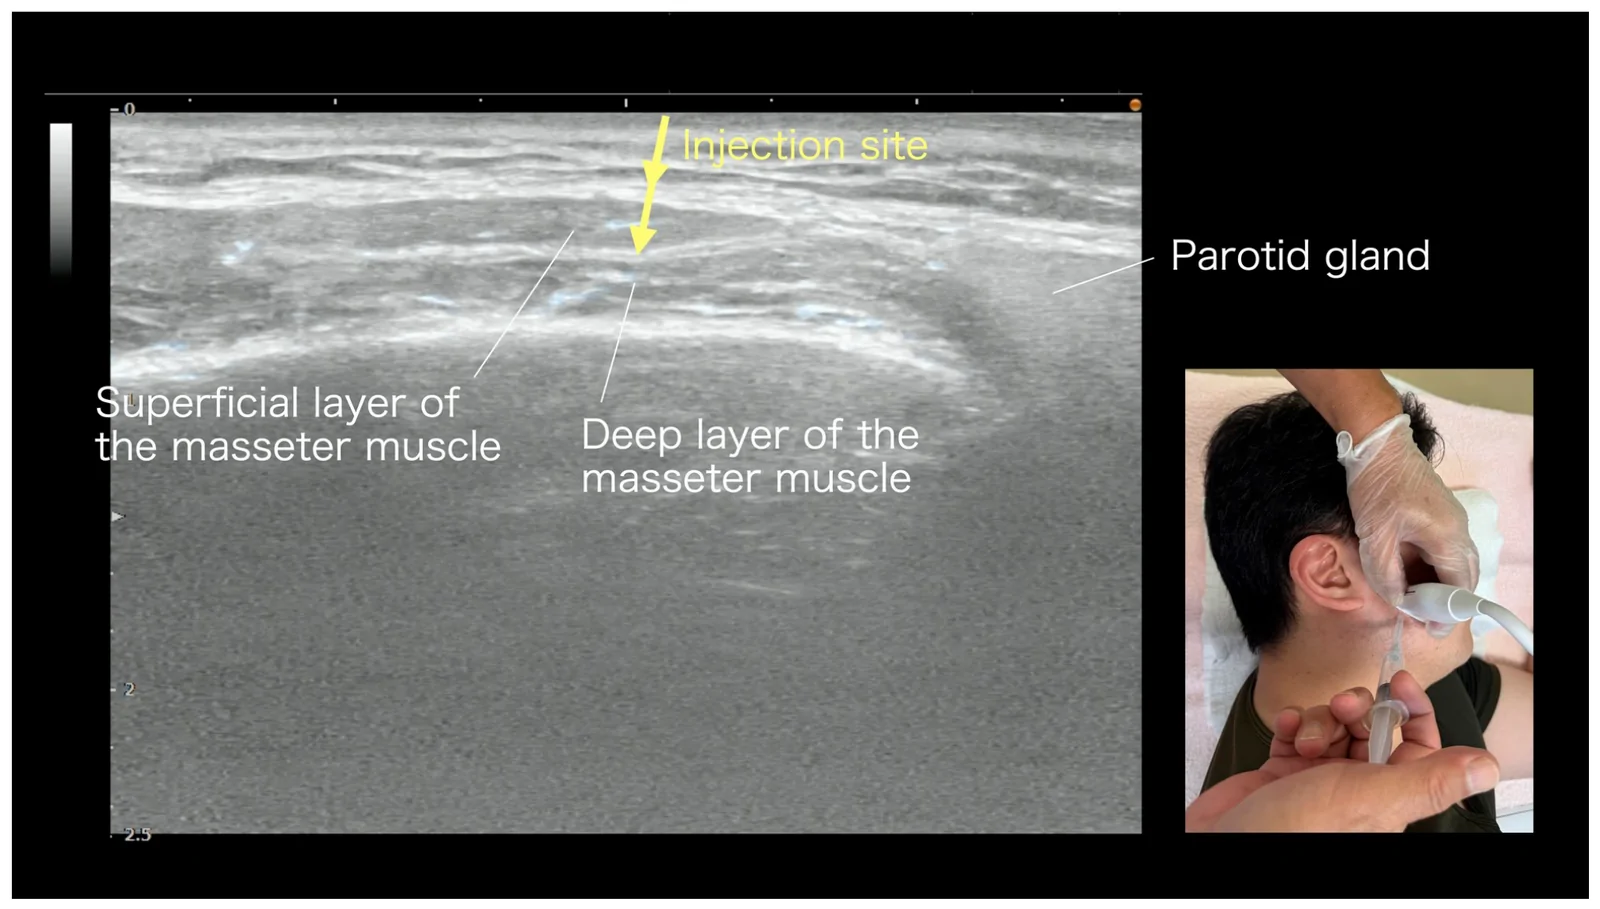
Masseter US-FHR (POINT 1). Ultrasound image: Superficial and deep layers of the masseter and the mandibular ramus are identified. The yellow arrow (injection site) indicates the plane between the superficial and deep epimysiums of the masseter. With a 27 G 38 mm needle, 1–2 mL of saline is injected via the out-of-plane approach. Color Doppler is used to confirm vascular structures before injection. The inset (bottom right) shows the patient position and probe placement.
